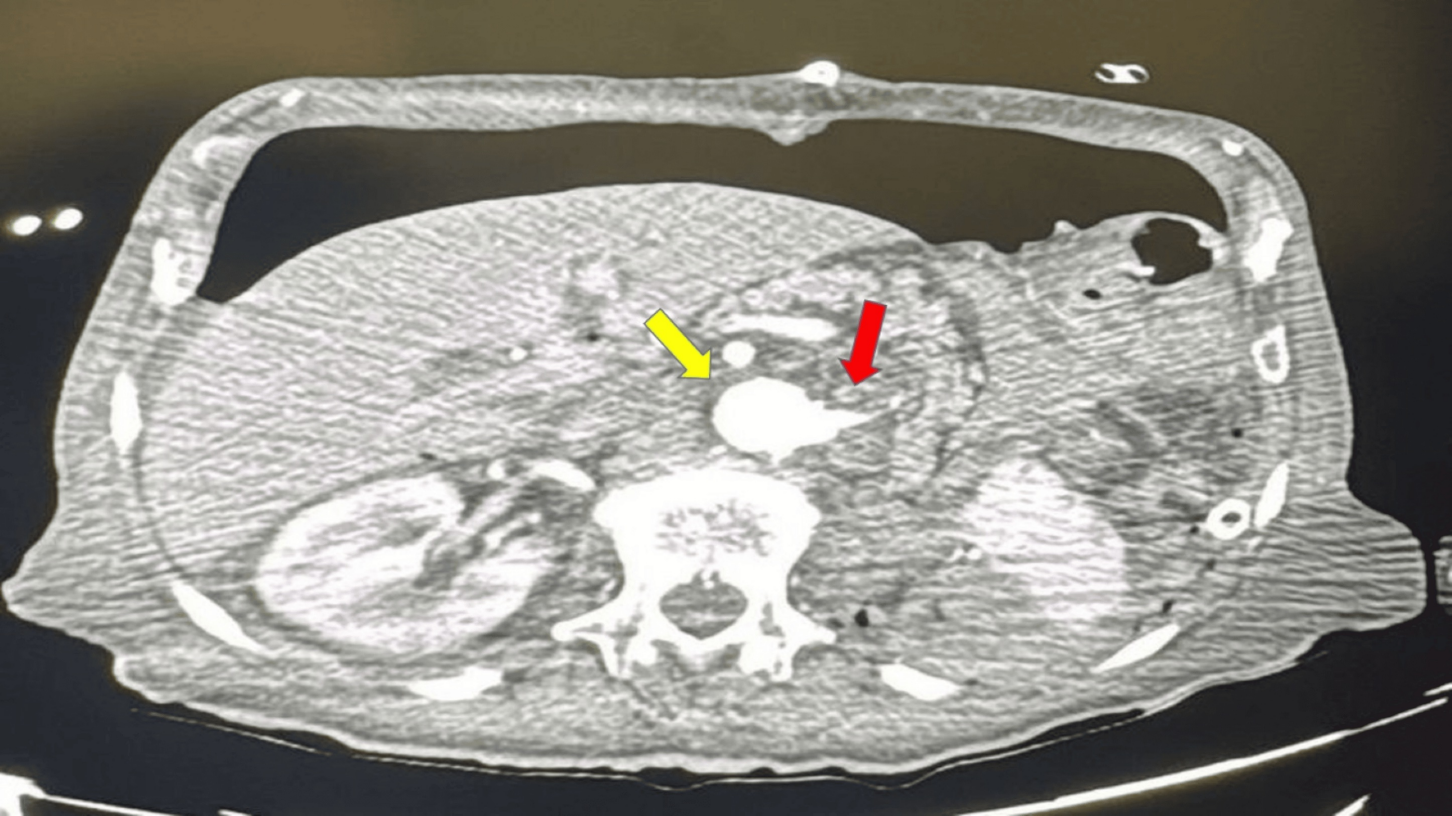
The CT angiography showed active bleeding from the left renal artery (red arrow) arising from the abdominal aorta (yellow arrow).
